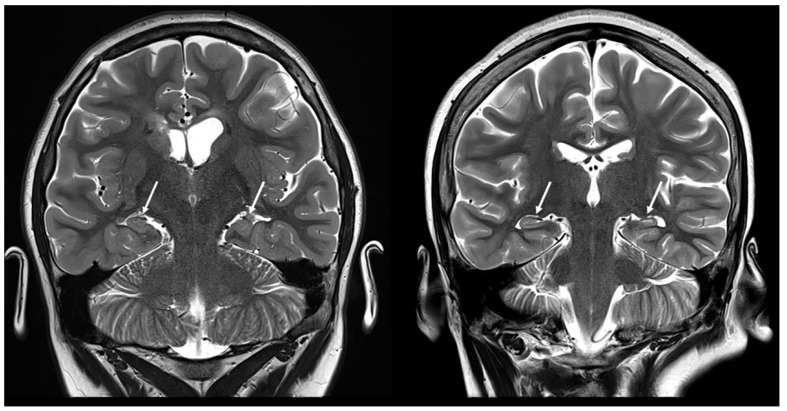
Coronal T2-weighted images demonstrating hippocampal abnormalities in two patients with tuberous sclerosis (10 and 12 years old, respectively).
